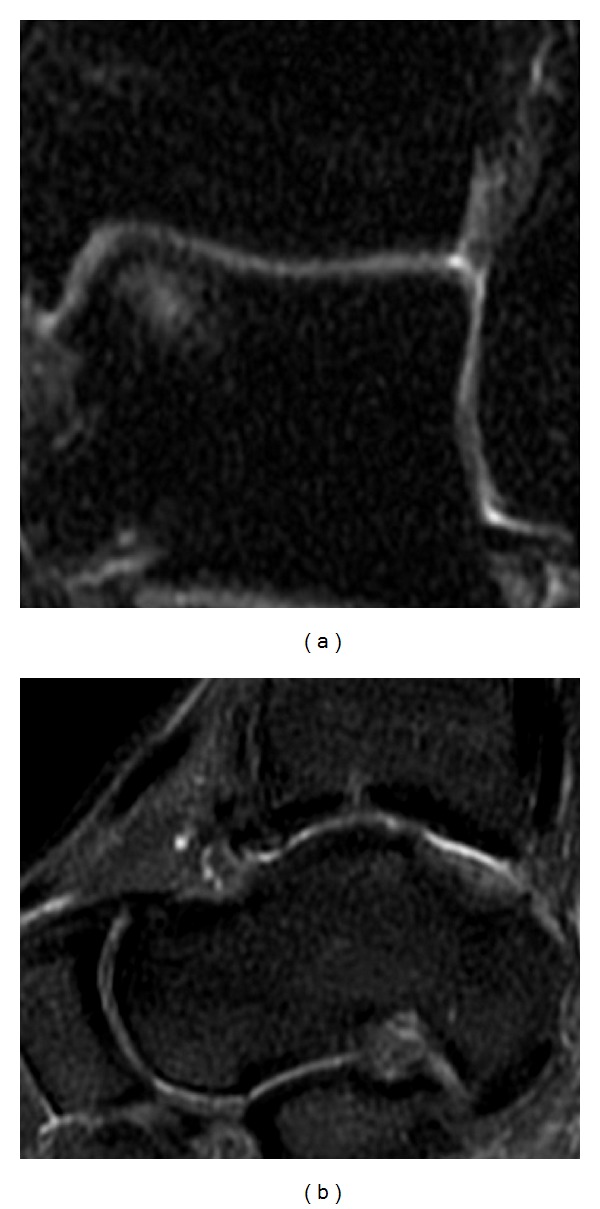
MRI performed 1 year after the second operation, coronal (a) and sagittal (b) views. There are no signs of the previous subchondral cyst. The MOCART scale demonstrates that the defect is completely filled.
